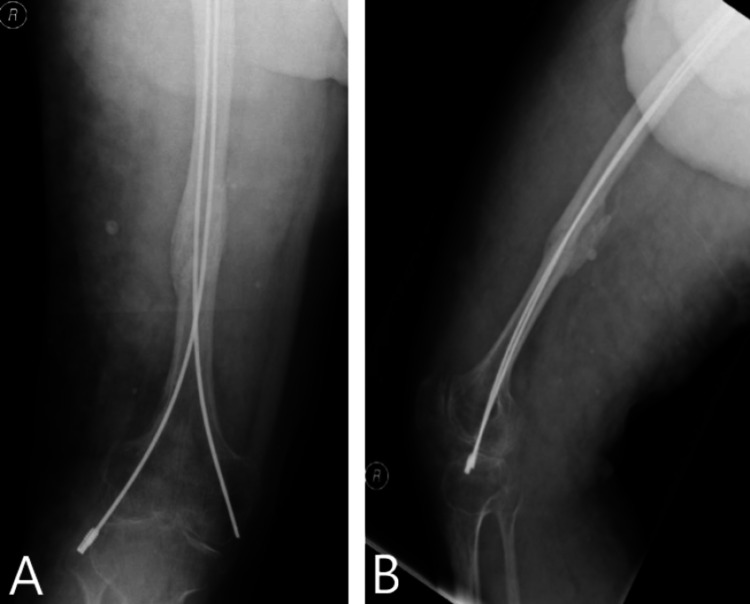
Radiographs showing radiological union of fracture with anteroposterior (A) and lateral (B) views
